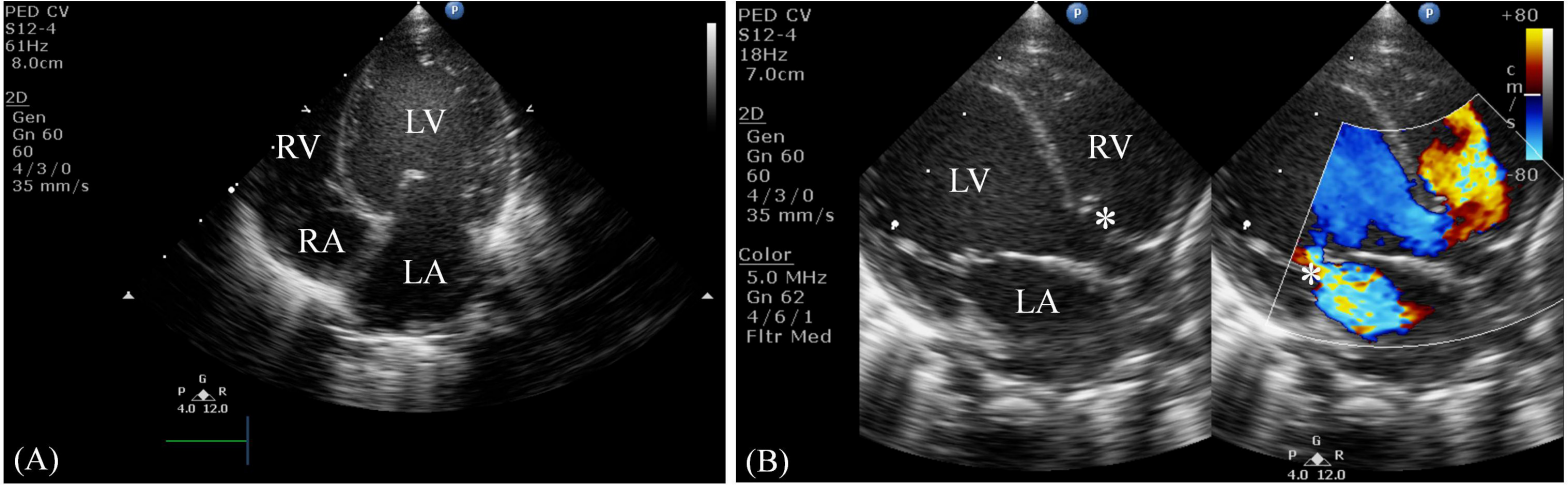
Two-dimensional echocardiogram. (A) This apical four-chamber view shows dilation of the left ventricle and atrium. (B) This parasternal long-axis view demonstrates a non-restrictive perimembranous-outlet type VSD sized 0.471 cm (indicated by the left white asterisk) and moderate mitral valve regurgitation (indicated by the right white asterisk). LV, left ventricle; RV, right ventricle; LA, left atrium; RA, right atrium.
